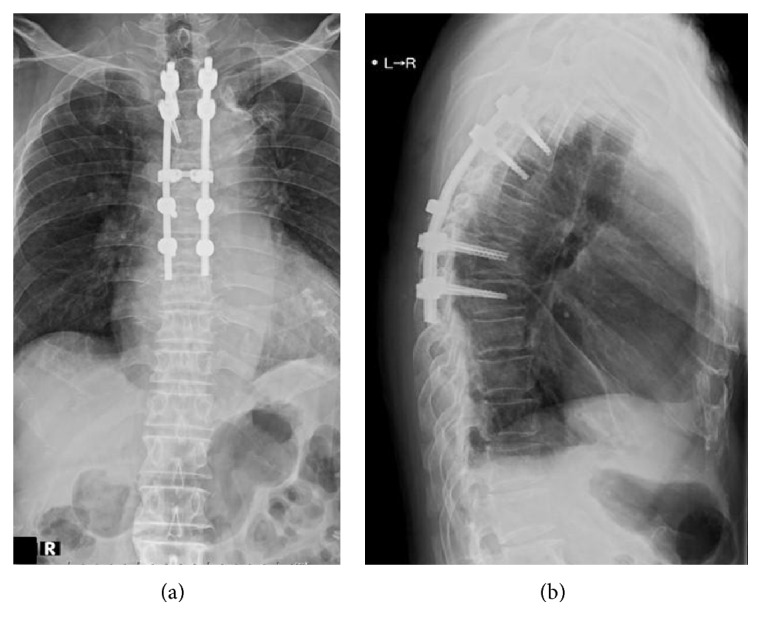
(a) Anteroposterior and (b) lateral radiographs show T3–8 posterior spinal segmental instrumentation and fusion.
